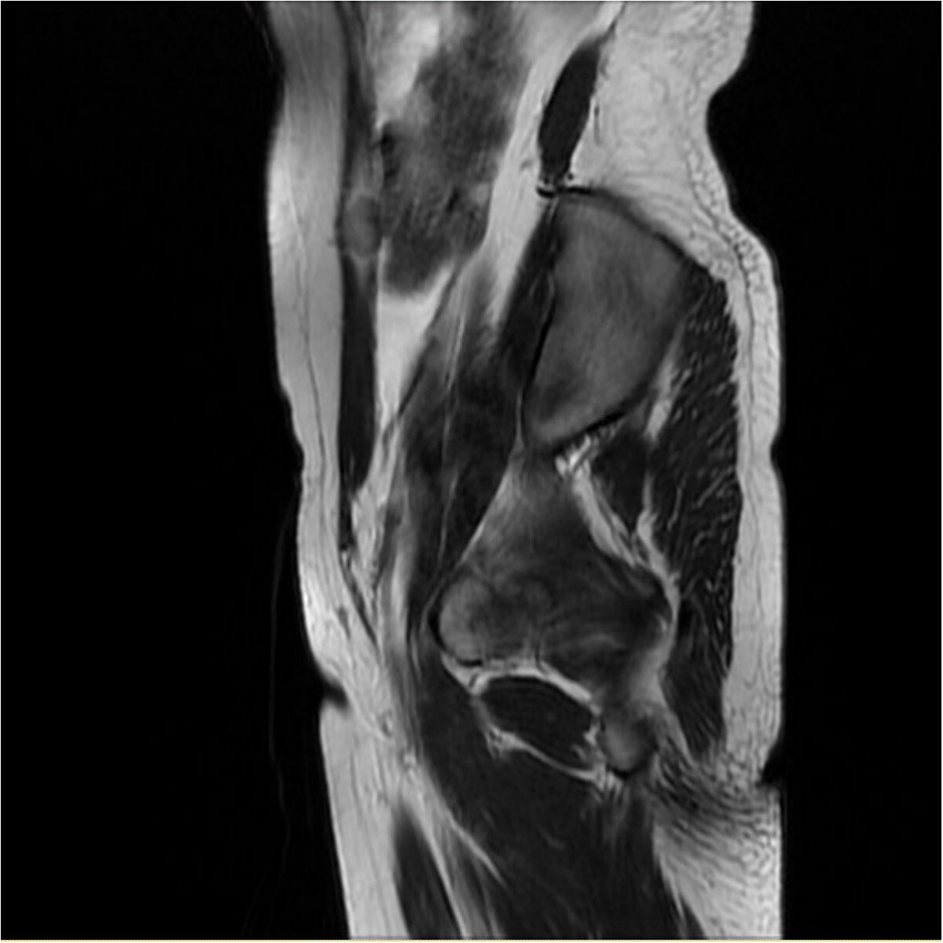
Sagittal MRI showed a nodule measuring 1.2 cm × 1.9 cm within the right rectus abdominis (Case 13).
